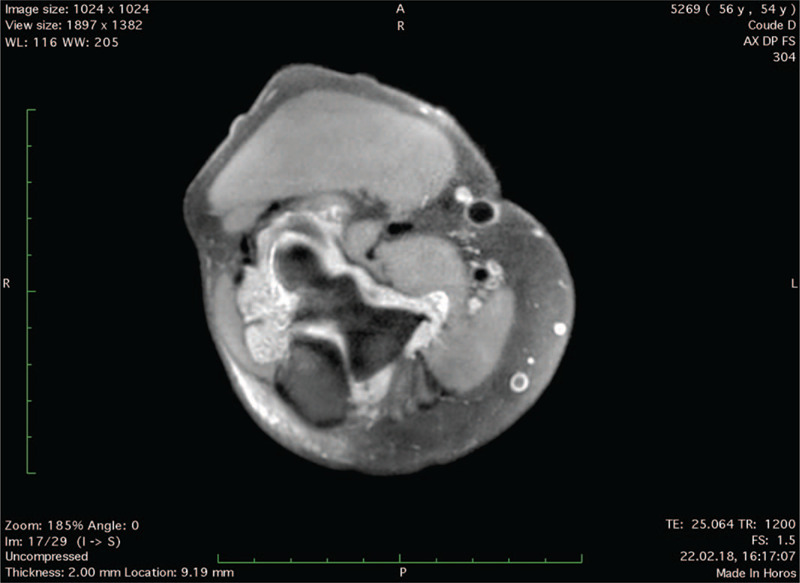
MRI DP FS sequence axial view of the right elbow showing intra-articular LA villi.
